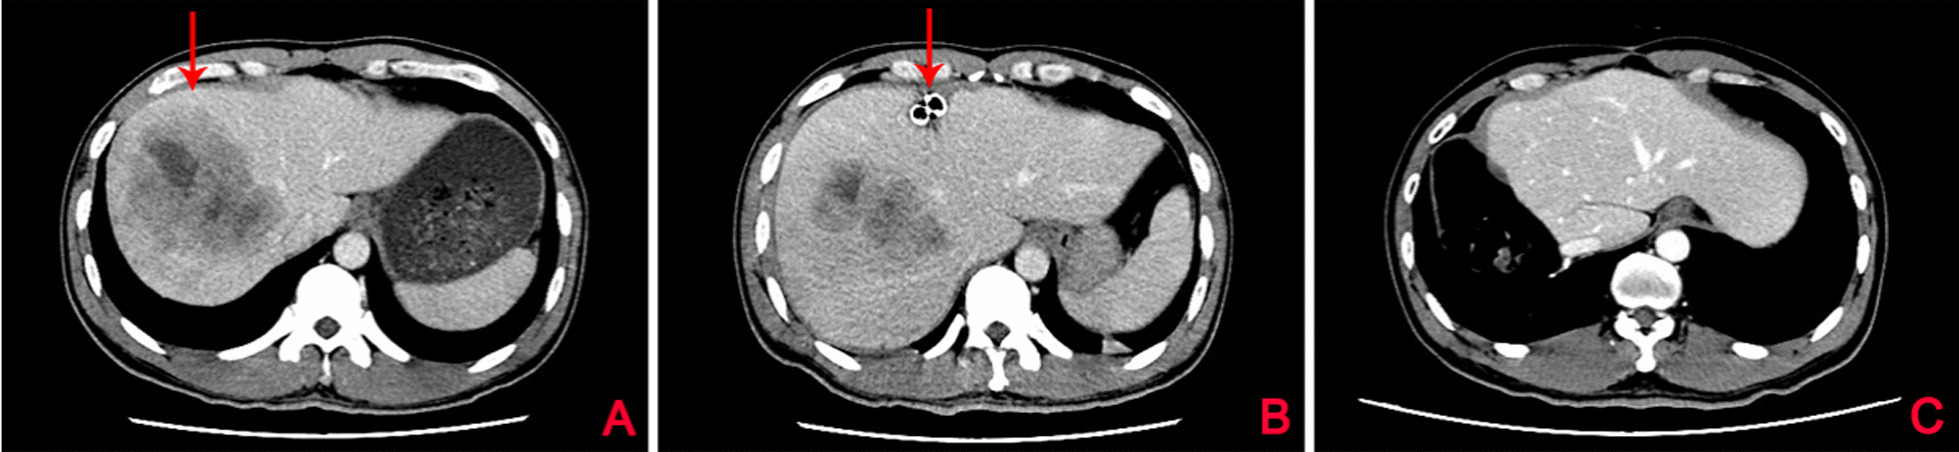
CT scan before and after ALPPS. A Patient’s preoperative CT scan. The red arrow pointed to the tumor, which was located in the right liver; B CT scan performed on the 3 d after the first stage of ALPPS. The red arrow indicated where the liver was dissected in the first stage procedure; C CT scan of the patient 3 months after the second stage of ALPPS
